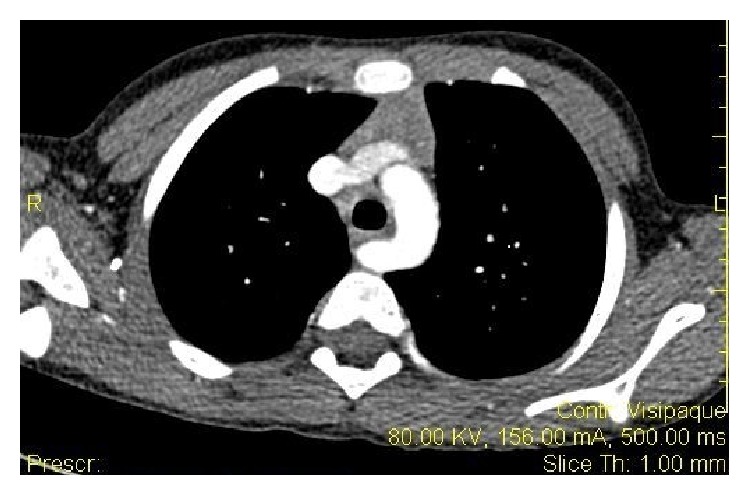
Angio-computed tomography*: demonstrating the aberrant right subclavian artery compressing the esophagus.
